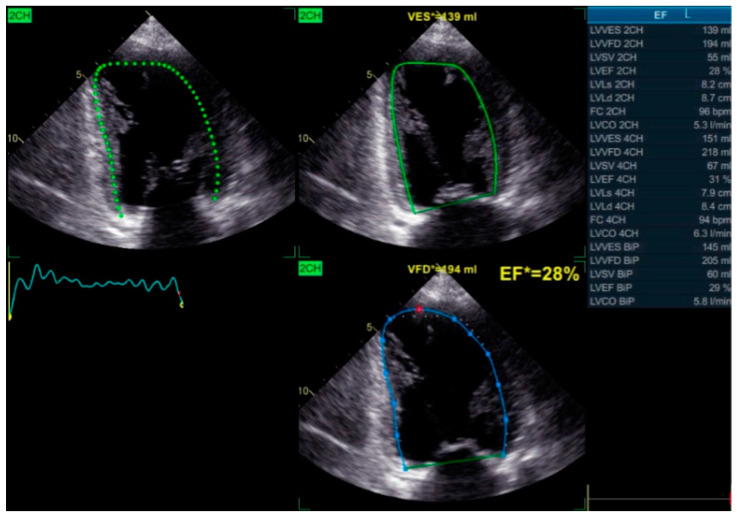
Biplane Simpson’s method echocardiographic assessment showing a reduced ejection fraction, indicating severe left ventricular systolic dysfunction.
